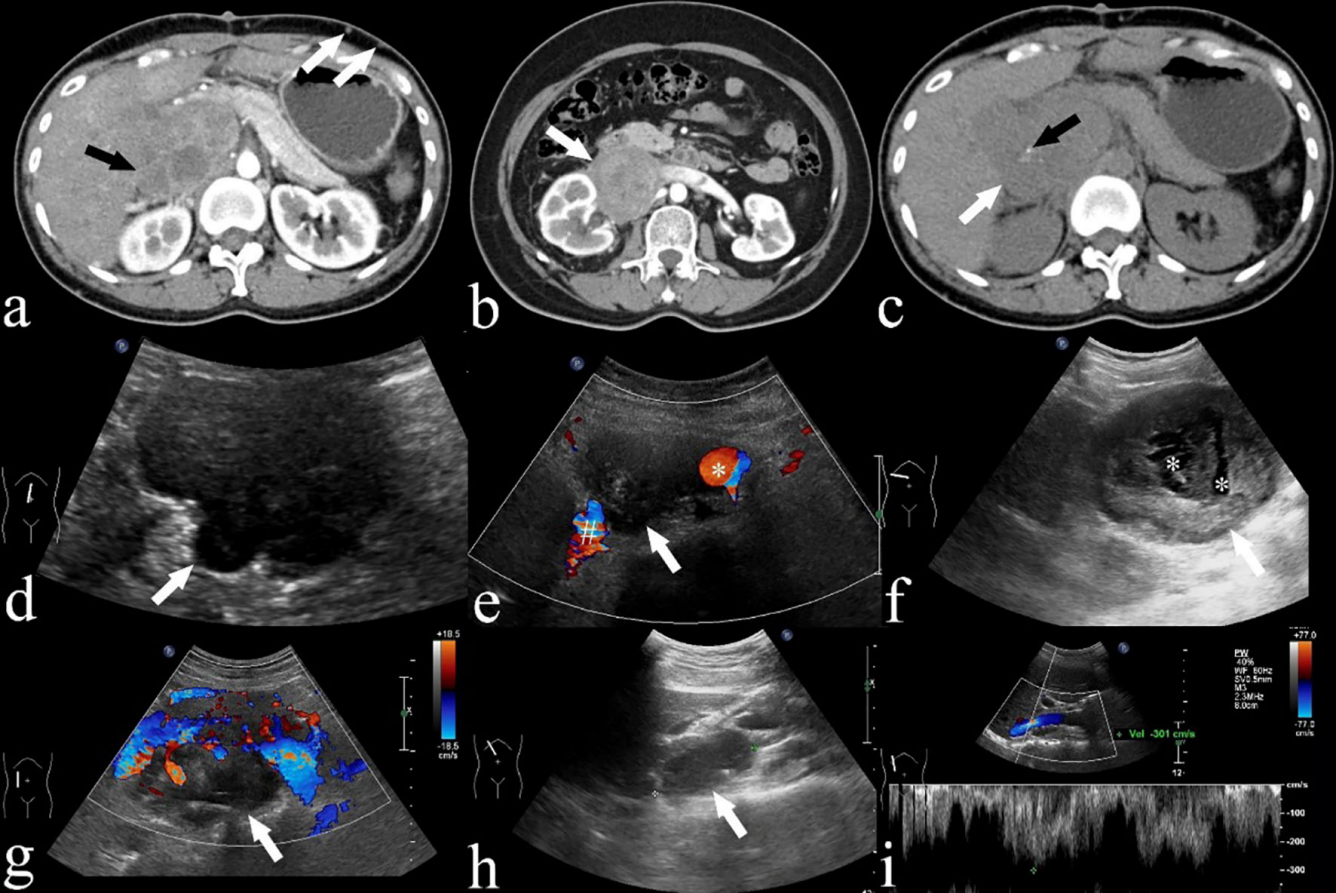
(A) A CECT of a 42-year-old woman (case 18) demonstrating an IVC LMS in segment II of the IVC (black arrow), distending the IVC. Despite being asymptomatic, the patient presents with extensive collateral formations (white arrows). (B) A CECT image of a 53-year-old woman (case 12) displaying a low attenuation mass (white arrow) extending into the right renal vessel and involving segments I and II. (C) A CECT image of a 42-year-old woman (case 18) demonstrating a low attenuation mass (white arrow) with punctate calcification (black arrow). (D, E) Ultrasound images of a 68-year-old woman (case 6) depicting a lobulated hypoechoic mass (white arrow) with extension into IVC (#) and aorta (*). (F) Ultrasound image of a 68-year-old woman (case 7) exhibiting an oval hypoechoic mass (white arrow) with anechoic areas suggesting necrosis (*). (G) Ultrasound image of an 80-year-old man (case 11) displaying an oval hypoechoic mass (white arrow) extending into IVC. (H, I) Pulsed wave Doppler images from a 34-year-old female (case 20) reveal high velocity flow in the IVC due to tumor compression.
